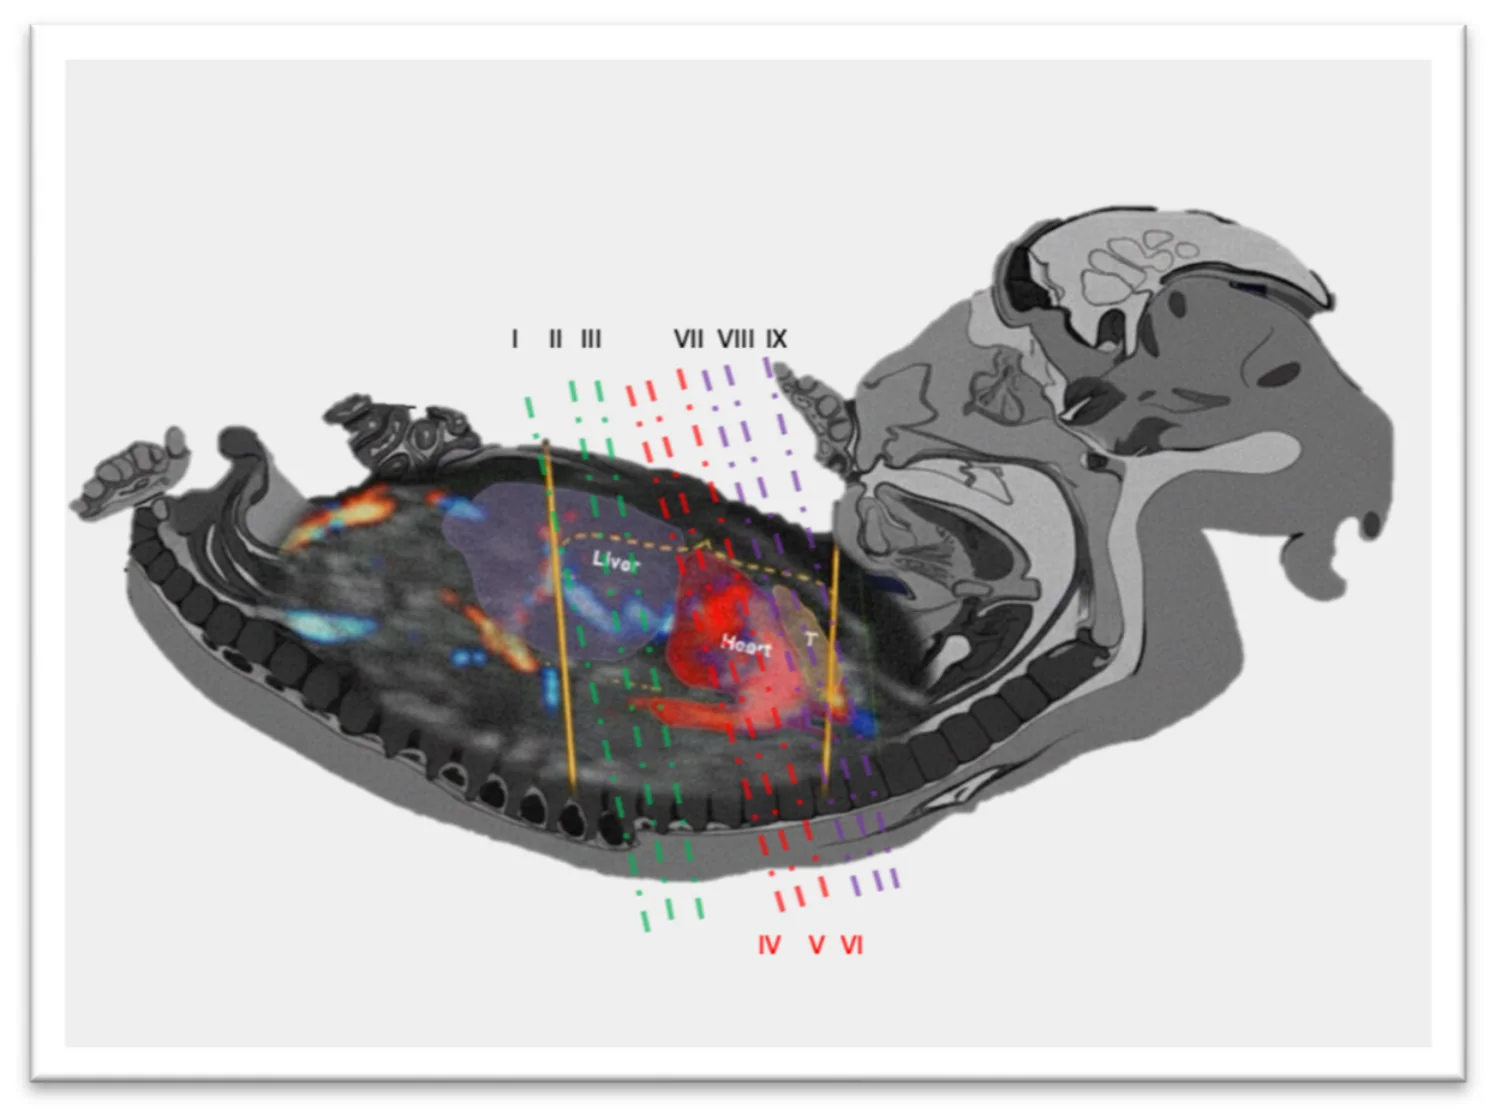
Sagittal representation of the Extended Fetal Cardiovascular System (EFCS) and distribution of the nine standardized axial ultrasound views of the Cardiovascular System Sonographic Evaluation Algorithm (CASSEAL) in a 12-week fetus (CRL ≈ 60 mm). Green indicates the infracardiac territory, red the cardiac territory, and purple the supracardiac territory.
